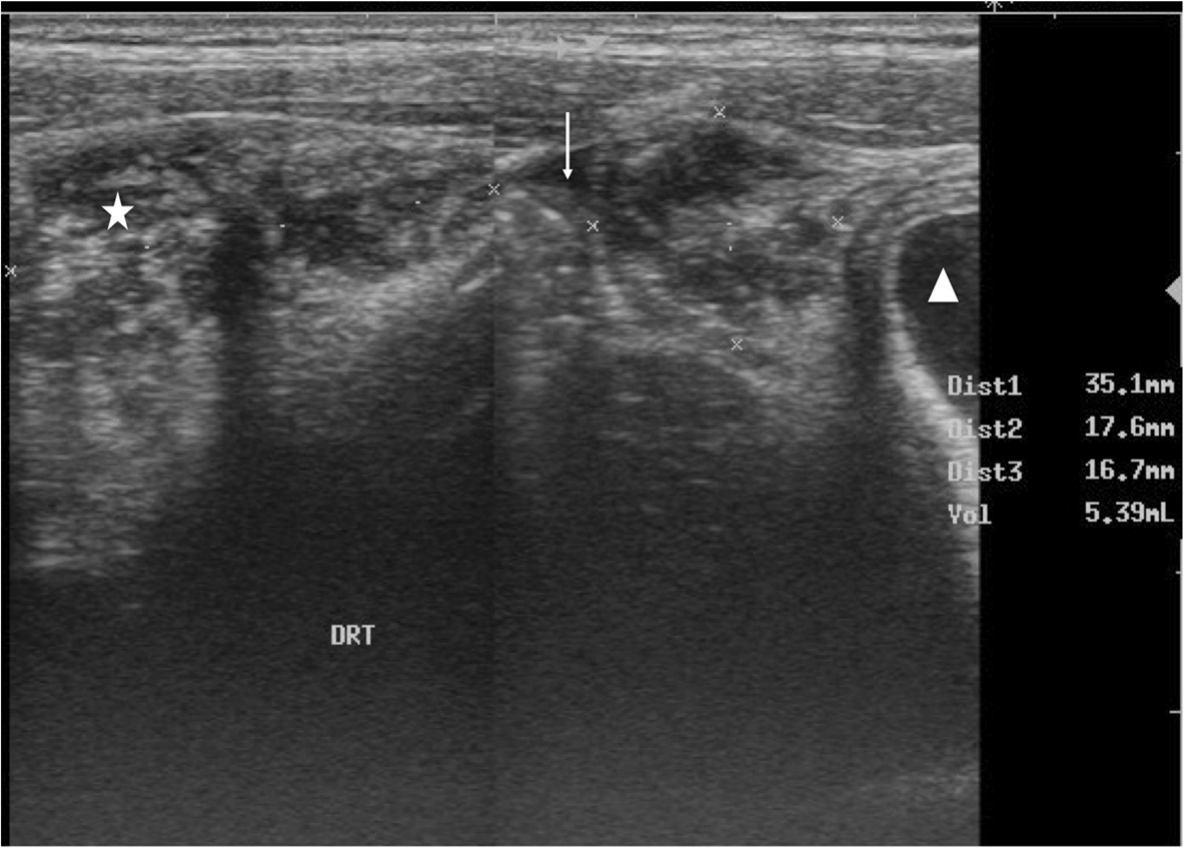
Ultrasound image of a longitudinal and b transverse section of the right iliac fossa. Adjacent to the bladder (triangle) there is a heterogeneous echogenic appearance of the psoas muscle (star) due to a hematoma associated with a discrete liquid collection (arrow)
